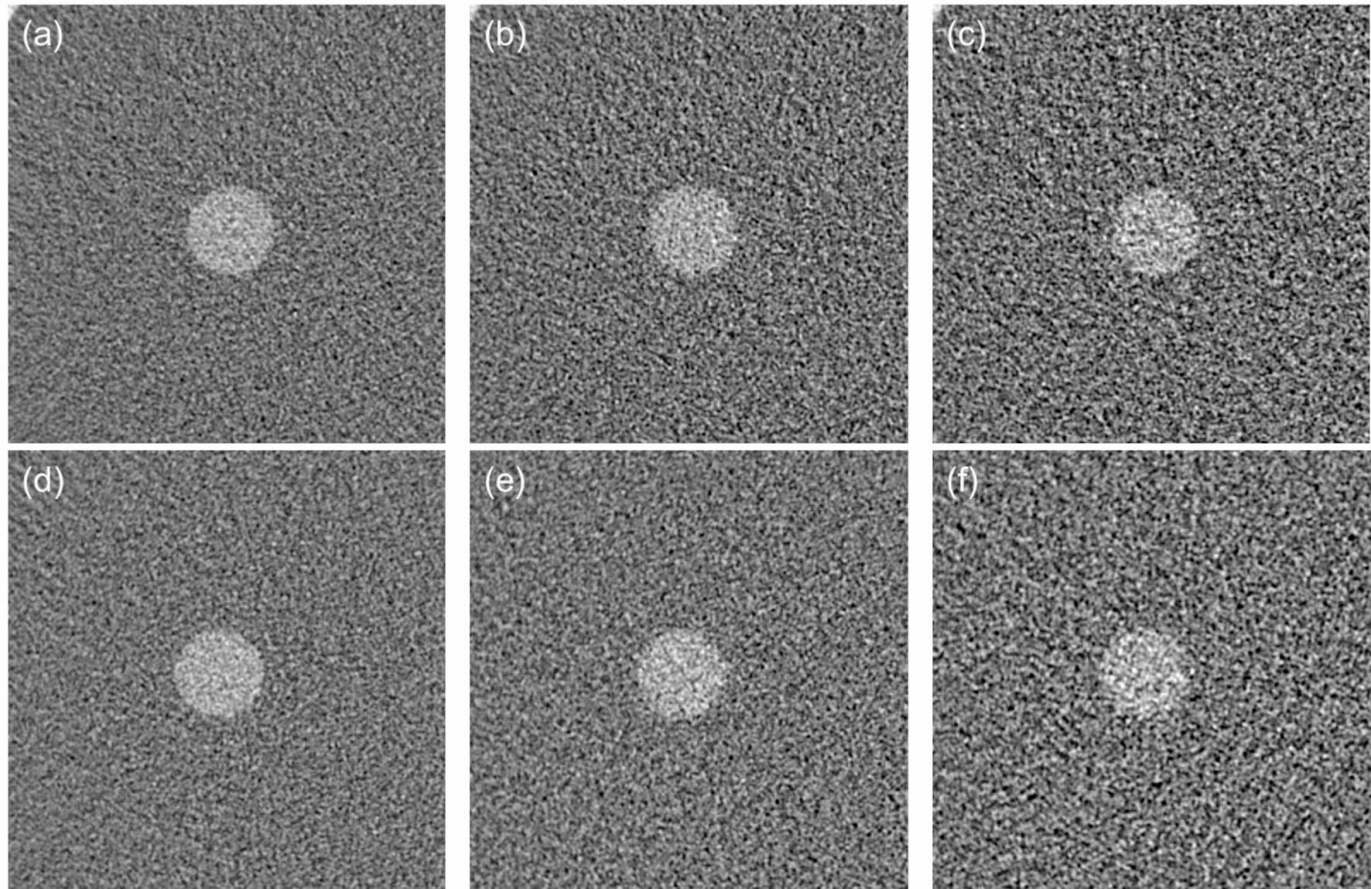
Comparison of virtual monoenergetic images at 40 keV of the rod with 2 mg iodine/mL. These images were obtained at the volume CT dose indices of a 15 mGy, b 7.5 mGy, and c 3 mGy for PCD CT; d 15 mGy, e 7.5 mGy, and f 3 mGy for DECT. The window width/level was set at 680/100
